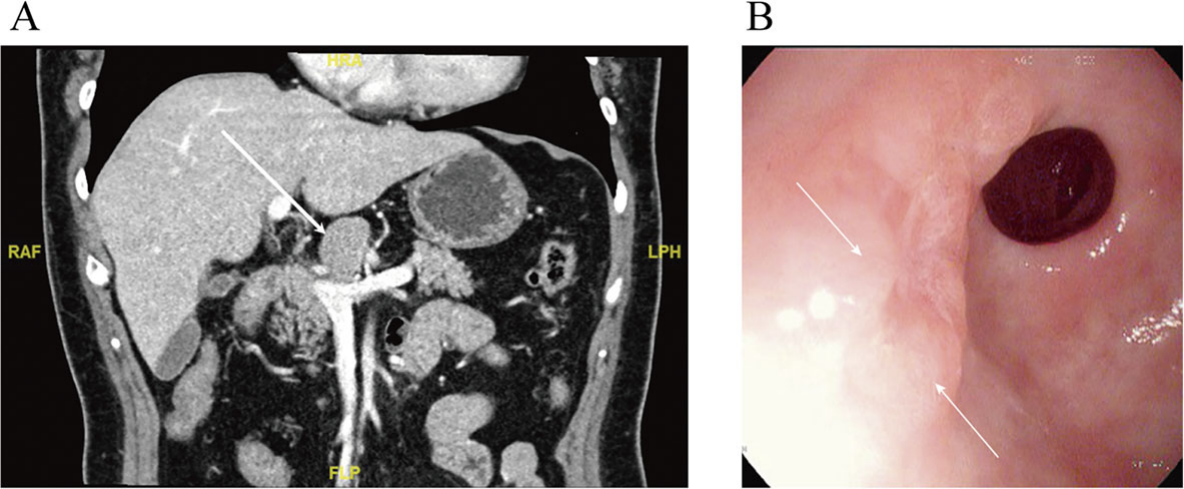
The last preoperative tests. a The latest contrast-enhanced abdominal CT. Stable solitary nodule (32 mm × 25 mm), labeled by the white arrow, without other abnormal findings. b The retaken gastroscopy found two bumps with mucosa erosion near the pylorus on the anterior gastric antrum, and a biopsy was taken around this area
